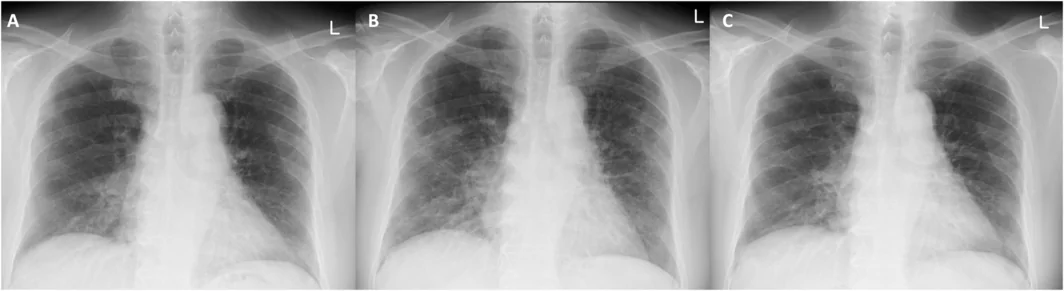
Serial chest radiographs. (A) Three months before presentation, normal findings. (B) At admission, decreased radiolucency in the bilateral middle and lower lung fields. (C) At 4‐month follow‐up, marked resolution of the opacities.
